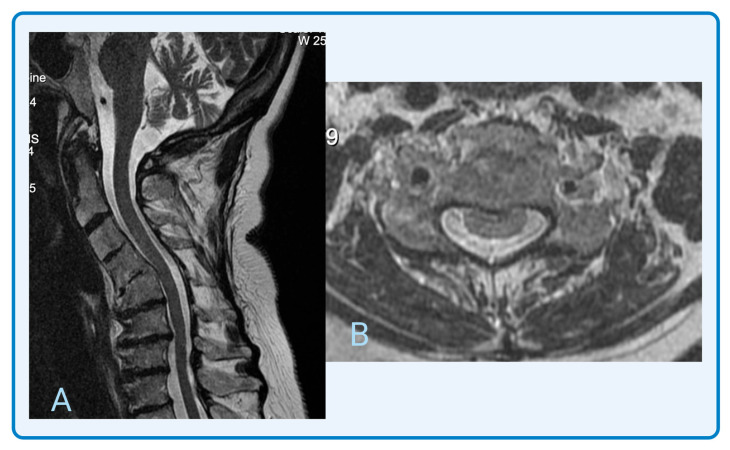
MRI cervical spine in sagittal (A) and transverse (B) views of a 65-year-old female diagnosed with DCM from the senior author’s clinical practice. This patient underwent surgical correction, resulting in severity improvement from Nurick grade IV to grade II.
